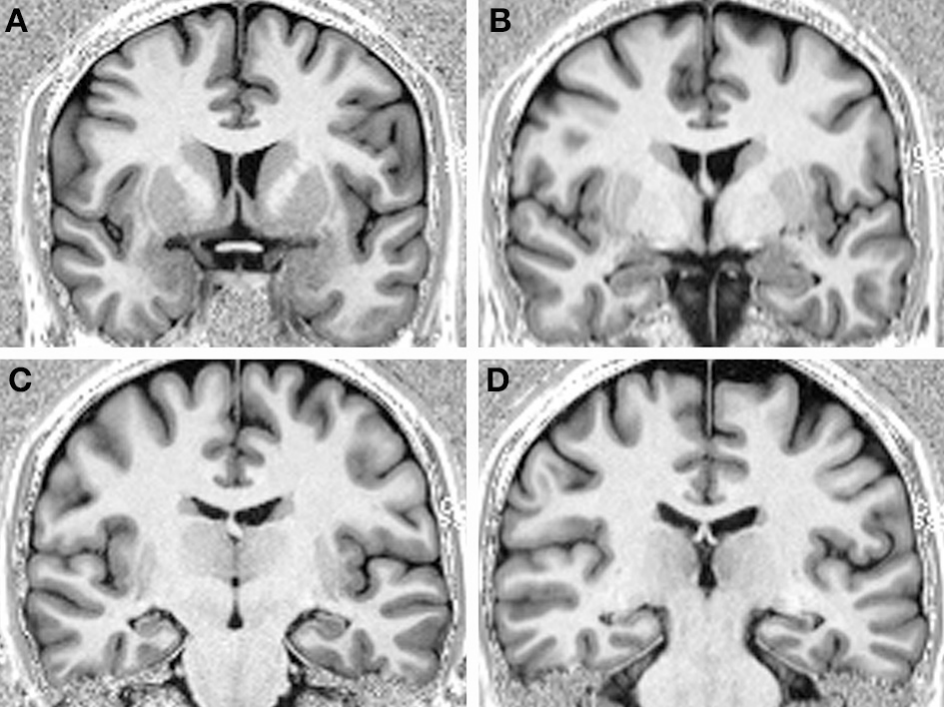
T1-weighted MRI images of P9's brain performed 3 years after the onset of pathology showing bilateral hippocampal atrophy, and no obvious signs of pathology in the surrounding cortical areas, from rostral (A) to caudal (D).
